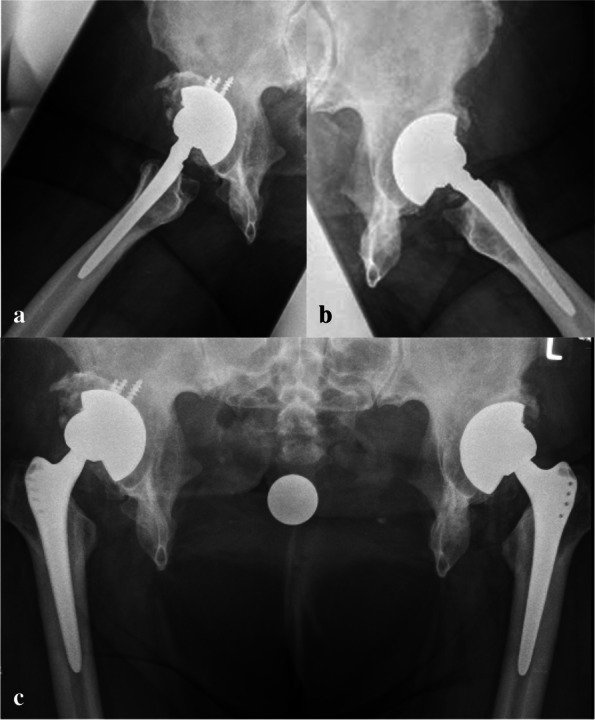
a, b) 9 years postoperative radiographic control demonstrated no signs of loosening of the acetabular or femoral component; c) 9 years postoperative radiographic control in lateral approach evidenced no signs of loosening of the implants
